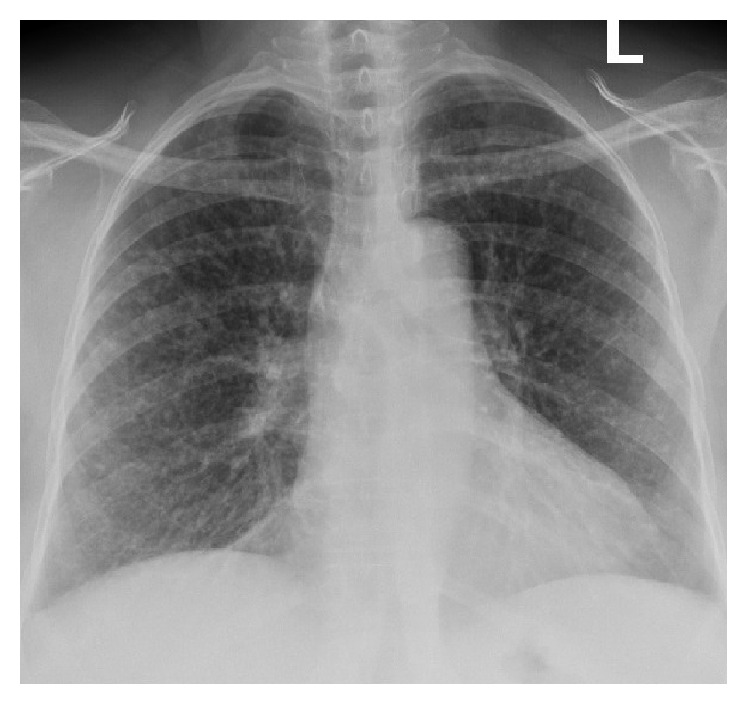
Chest radiograph demonstrating diffuse micronodular densities consistent with a miliary pattern.
